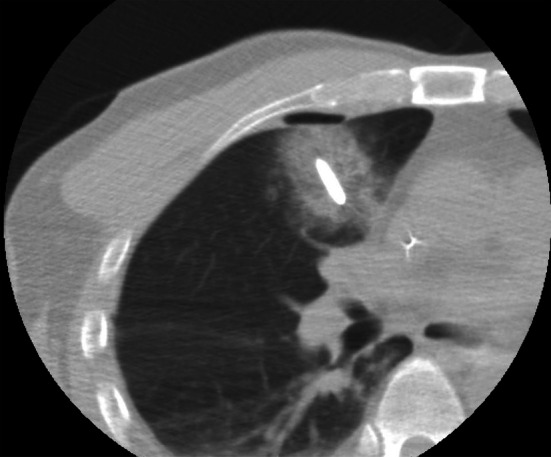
Computed tomography images of percutaneous cryoablation of the tumor located in segment 5 of the right lung.
